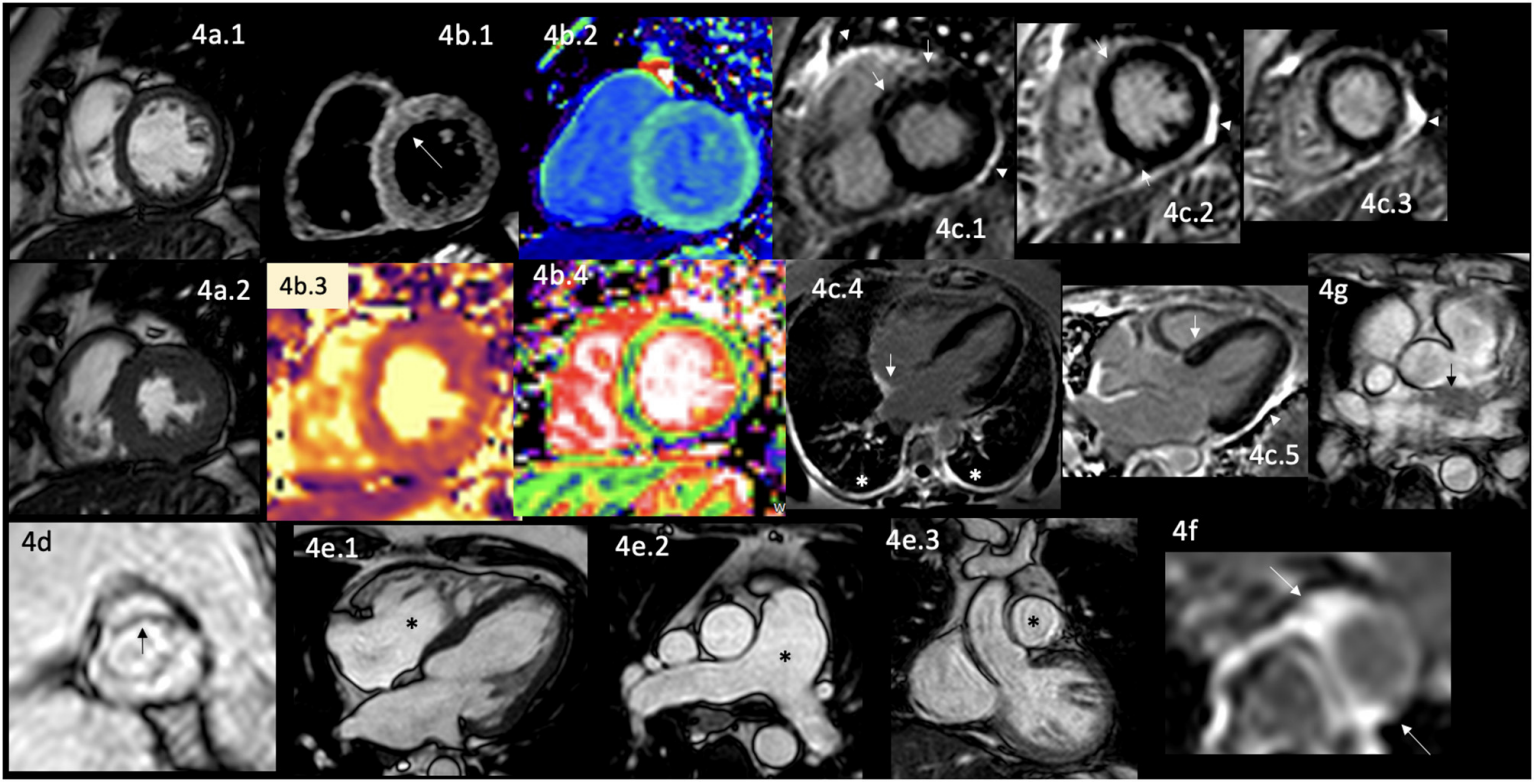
CMR findings in AR. A panel figure demonstrates varying findings from a comprehensive CMR study at a 3.0 Tesla scanner of RA with PH and arrhythmias. (a) SSFP sequence still cine images in short-axis view at the mid-ventricular level in end-diastole (a.1) and in end-systole (a.2) show normal global and regional left ventricular functions, mass, and wall thickness, LVEF 64% and moderately reduced systolic global RV function with global hypokinesia and mild hypertrophy (RV mass index 95 g/m^2^), RVEF 44%. (b.1) T2-W STIR sequence in short-axis view at a mid-ventricular level showing mildly increased signal intensity in the anterior, anteroseptal, and inferoseptal segments (arrow) with a myocardial/skeletal muscle ratio of 2.4 suggesting mild myocardial edema. (b.2) T1 mapping with a native T1 of 1,212 ms (normal), (b.3) T2 mapping with a T2 time of 42 msec (upper limit of normal) and (b.4) the ECV map corresponding to an elevated 52%. (c) LGE PSIR sequence. (c.1–3) Short-axis views at basal (c.1), mid (c.2), and apical (c.3) levels, and long-axis views in four-chambers (c.4) and three-chambers (c.5) projections. The arrows in these images show areas of focal fibrosis as midventricular LGE in the anteroseptal segment in (c.1,2) and the subepicardium of the anterior segment in (c.1) and subepicardial in the inferior segment in (c.2). (c.4) Shows LGE on the upper RA wall (arrow) and both-sided pleura (asterisks). (c.5) Shows mid-wall LGE on the anteroseptal segment. Pericardial LGE is shown in (c.1–3,5) (arrowheads), consistent with pericardial inflammation in the absence of pericardial effusion. (d) Zoomed still cine image in SSFP sequence of the aortic valve showing cusps thickening (arrow) with a mildly reduced valvular area (1.57 cm^2^). (e.1–3) SSFP sequence still cine images in long-axis 4-chambers view (e.1) showing RV enlargement (asterisks), (e.2) true axial view at great vessels level showing a mildly enlarged PA (asterisks) confirmed in the corresponding coronal view (e.3). (f) Zoom LGE PSIR image of the descending aorta showing wall artery enhancement (arrows). (g) SSFP sequence still cine images in the true axial view show an isointense tissue between the aortic root and the LA, probably of a granulomatous origin (arrow). CMR, cardiovascular magnetic resonance; RA, rheumatoid arthritis; SSFP, steady-state free precession; LVEF%, left ventricular ejection fraction; RVEF%, right ventricular ejection fraction; T2-W STIR, T2-weighted short-tau inversion recovery; ECV, extracellular volume; T1-W, T1-weighted; LGE, late gadolinium enhancement; PSIR, phase-sensitive inversion recovery; PA, pulmonary artery; LA, left atrium.
